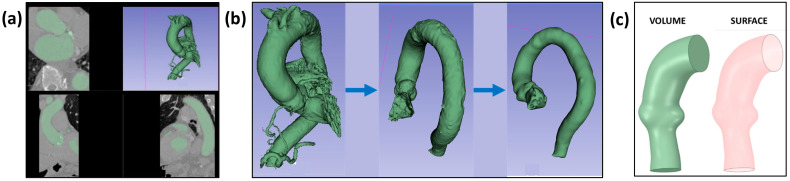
Aorta geometry generation (a) Patient-specific CT images, (b) 3D geometry segmentation using 3D Slicer software, and (c) final volume and surface geometries for FEA.
